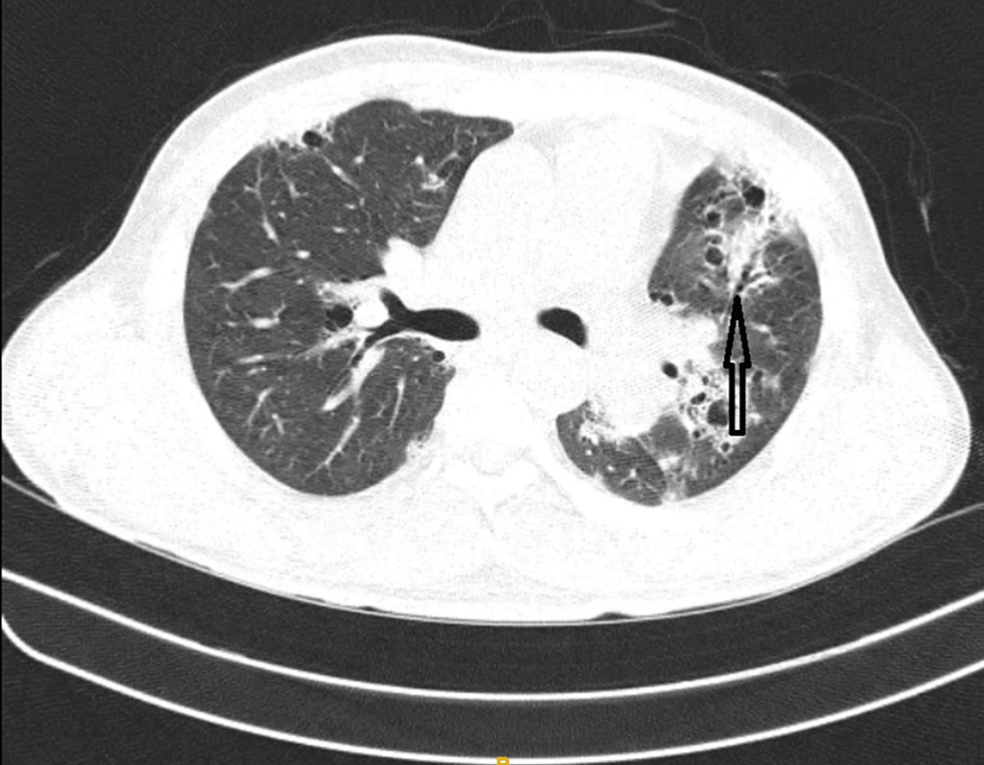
HRCT Showing Patchy Consolidation With Pleural Thickening (Black Arrow) HRCT: High-resolution computed tomography
